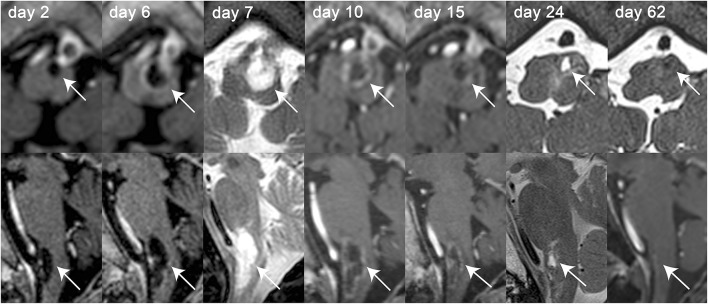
Axial (top row) and sagittal (bottom row) MR-images of the intra-axial cystic lesion (indicated by the white solid arrows) located in the left part of the medulla oblongata over the course of 2 months. MR-images were obtained on day 2, 6, 7, 10, 15, 24, and 62 after symptom onset as indicated on the single MR-images. Whenever available, T1-weighted contrast-enhanced MR-images were selected for both axial and sagittal images [day 2, 6, 10, 15, 62 (only sagittal image)]. For the other MR-sessions, either T2-weighted (day 7) or gradient-echo T1-weighted [day 24 and 62 (only axial image)] MR-images were selected. Over the course of disease growth [from 16 × 5 × 4 mm on day 2–25 × 11 × 9mm on day 10 (height × depth × width)] and contrast-enhancement (first noted on day 6) of the cystic lesion can be depicted. After treatment initiation with dexamethasone (day 9) and surgical preparation of the solid lesion (day 15), the size of the cystic lesion decreased continuously and could not be detected any more on day 62.
